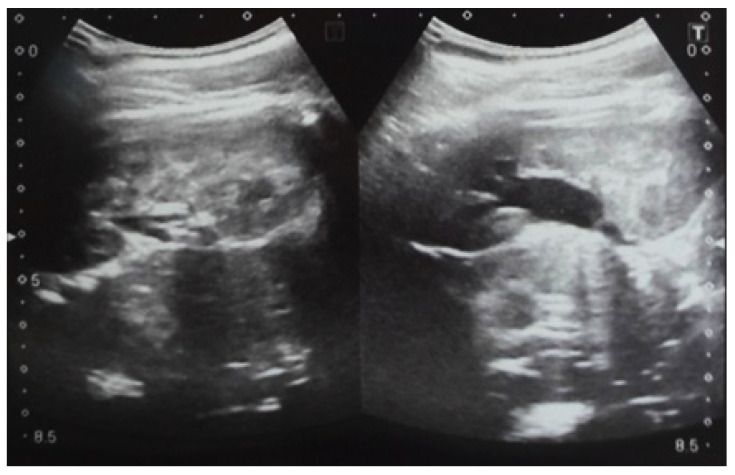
Postnatal urinary ultrasound. Hydronephrosis on the left.
